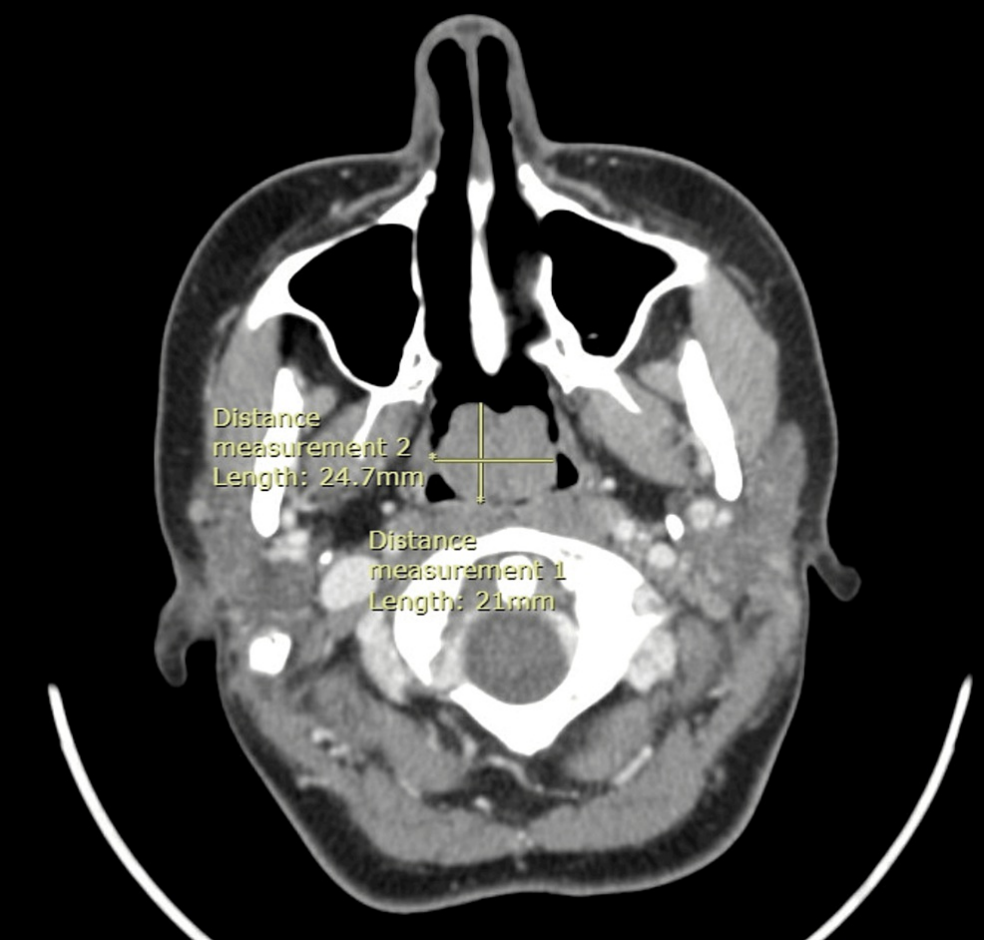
Axial CT scan The axial CT scan shows the nasopharyngeal mass with measurements of the size (approximately 2.47*2.1 cm).
